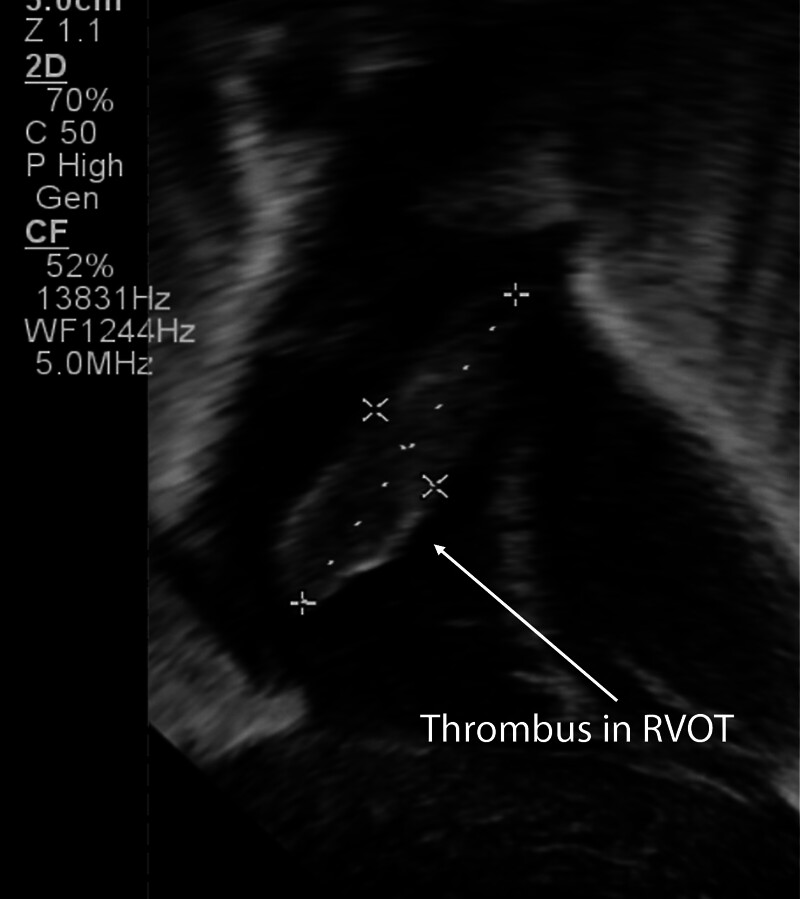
Echocardiogram day of life 29. Initial cardiac thrombus identified in the right ventricular outflow tract (RVOT).
